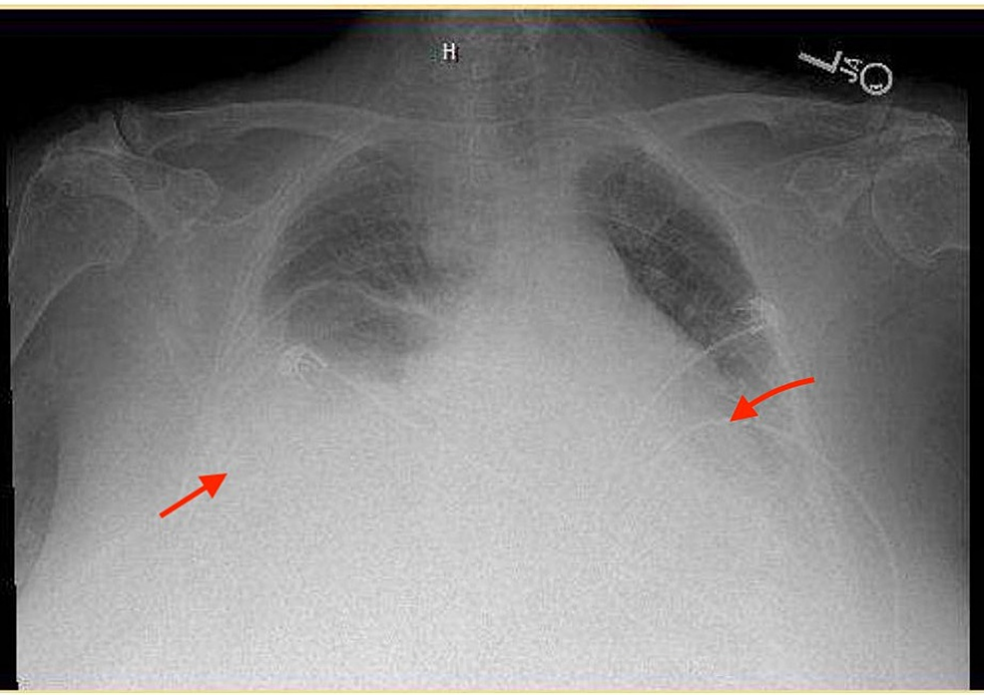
Chest X-ray PA view: moderate right pleural effusion, minimal left pleural effusion. PA, posteroanterior
